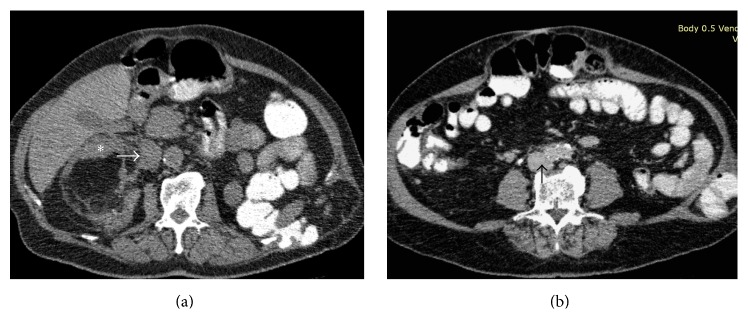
Axial CT images after embolization and ablation. (a) Unenhanced image shows that the angiomyolipoma is smaller, with relative shrinkage of the soft-tissue component (*∗*) in favor of the fat. The compression of the IVC (arrow) is now less striking. (b) Contrast-enhanced image (venous phase) shows disappearance of the caval thrombus (arrow).
